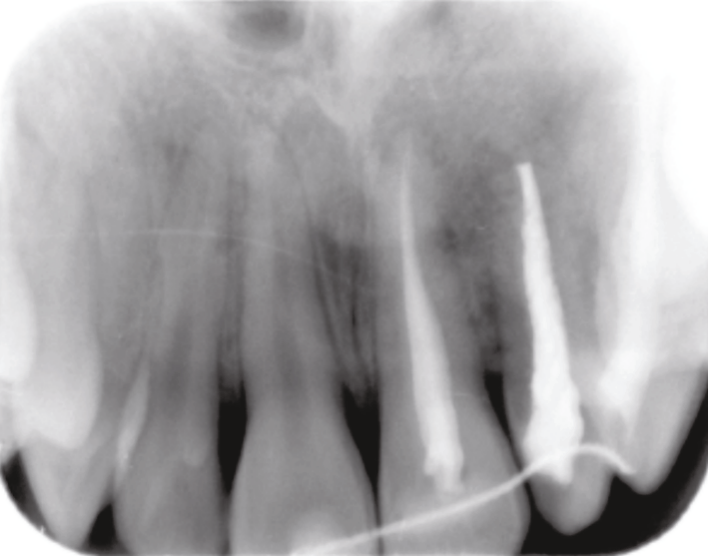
Healing at 6-month follow-up, X-ray.
